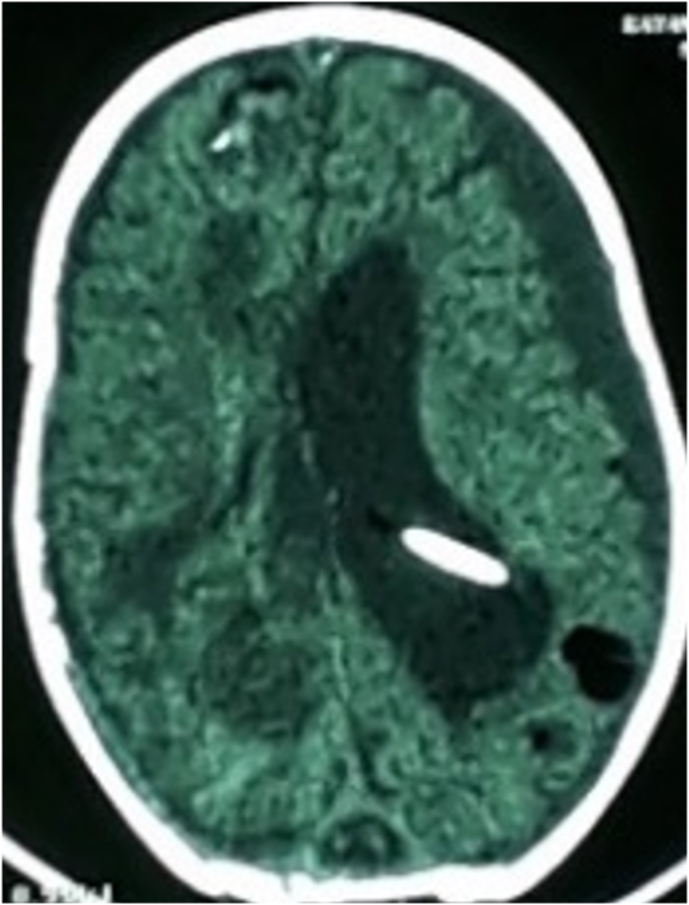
Head CT scan showing hydrocephalia with cerebral abscess.
